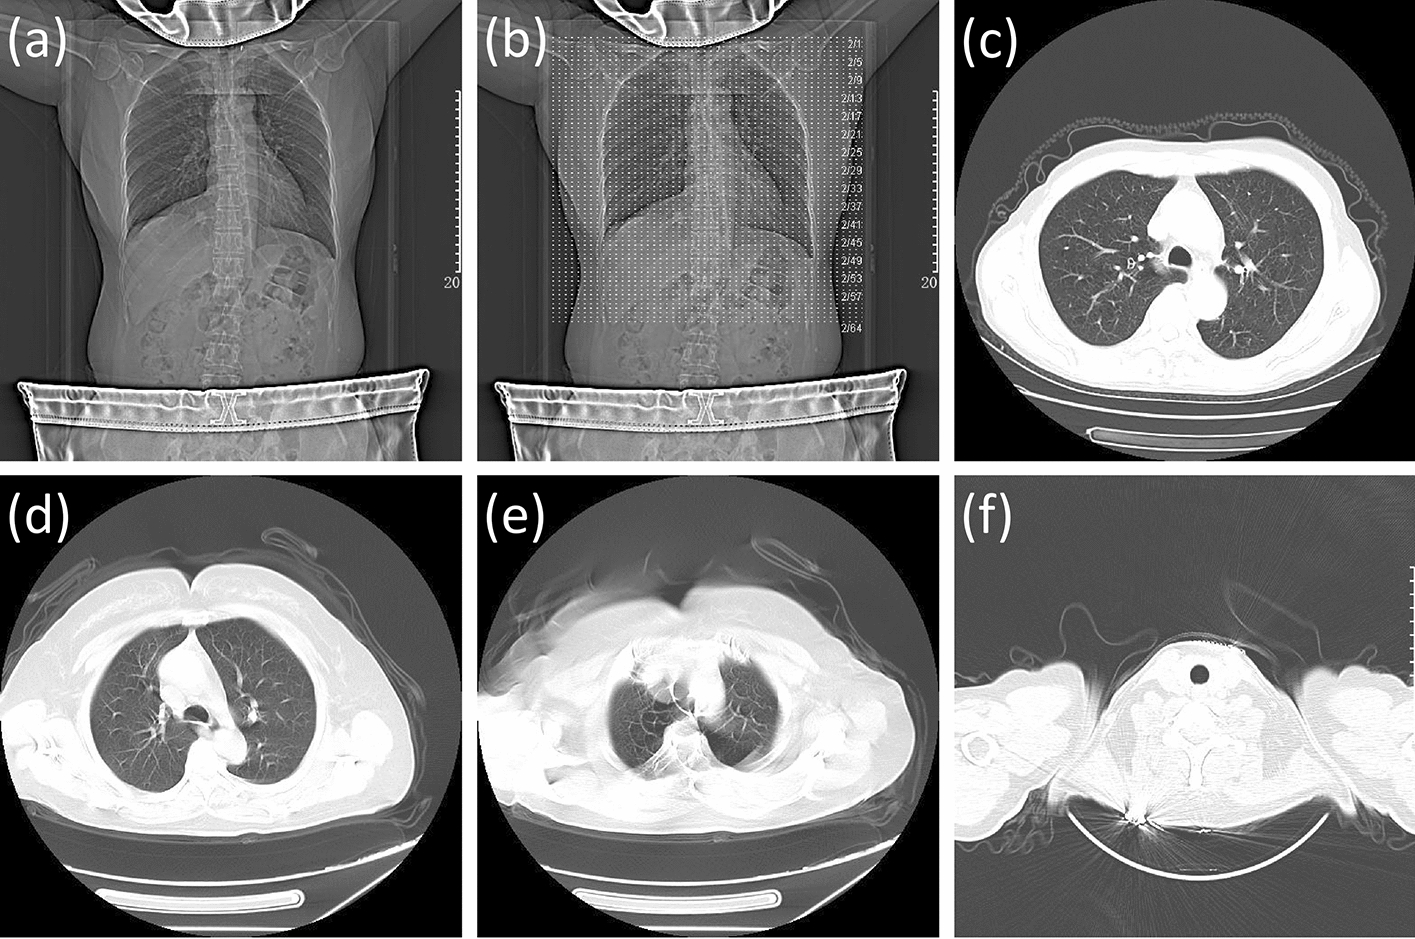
The results of patients who met and did not meet the requirements of filming. a Indicates that the patient raising arms above head, wearing radiation-protective products on neck and abdomen, and no metal objects while filming. b Is the CT image of a after overlaying the scan baseline. c Represents the patient filming at the prescribed scan FOV range, while d represents the patient with a portion of body outside the prescribed scan FOV range. e Indicates the presence of motion artifacts. f Indicates the presence of metal artifacts
